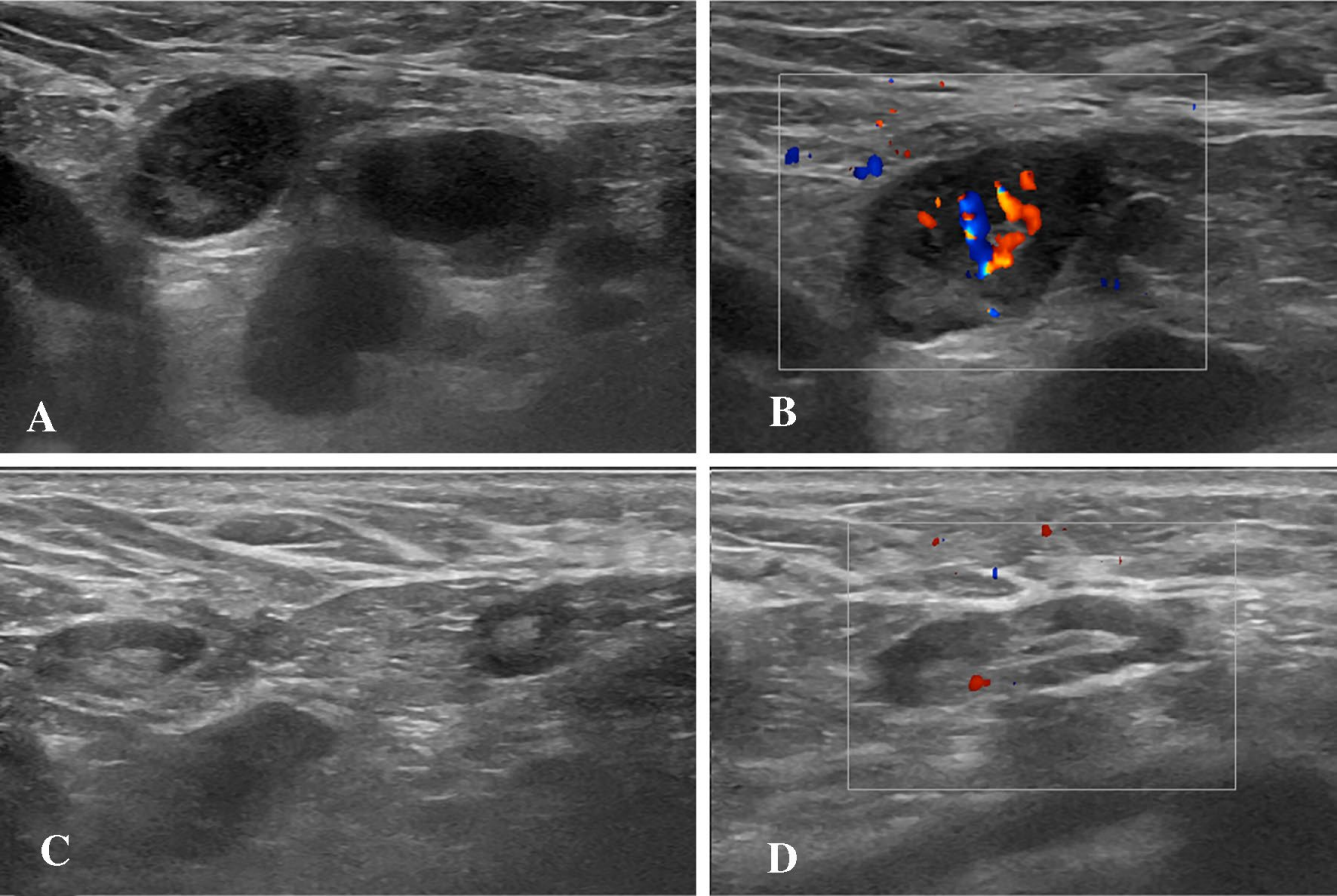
B-Mode (A) and color-Doppler (B) US examination performed 2 days after receiving the first dose of vaccine: multiple axillary lymph nodes (maximum diameter 15 × 10 mm) with poorly represented fatty hilum (A) and moderate vascularization (B) were found. B-Mode (C) and color-Doppler (D) US examination performed after 4 weeks. Although lymph node size remained stable, fatty hilum was restored (C) and vascularization reduced at color-Doppler (D)
